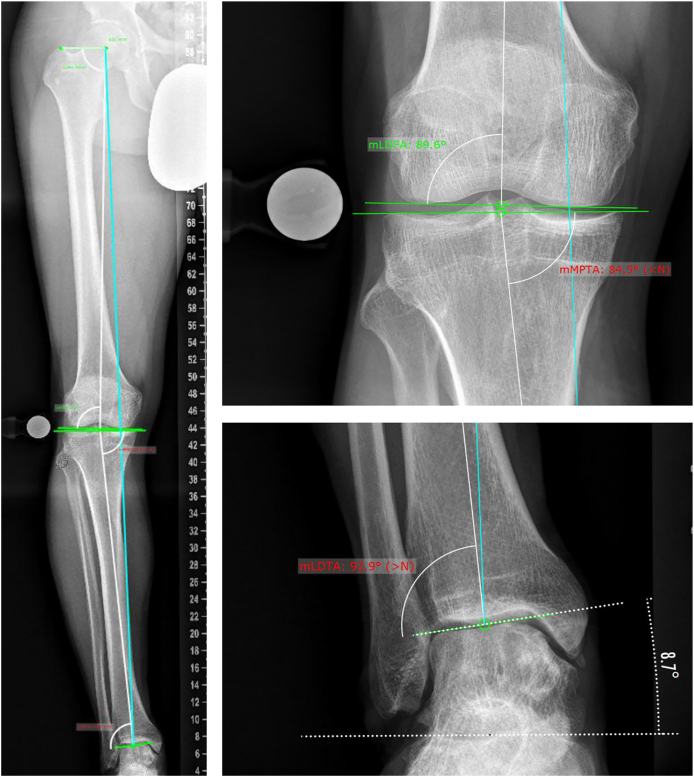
Standing whole-leg radiography demonstrating axial malalignment of the lower limb with varus deformity at the knee and upper ankle joint, accompanied by pathological talar inclination.
